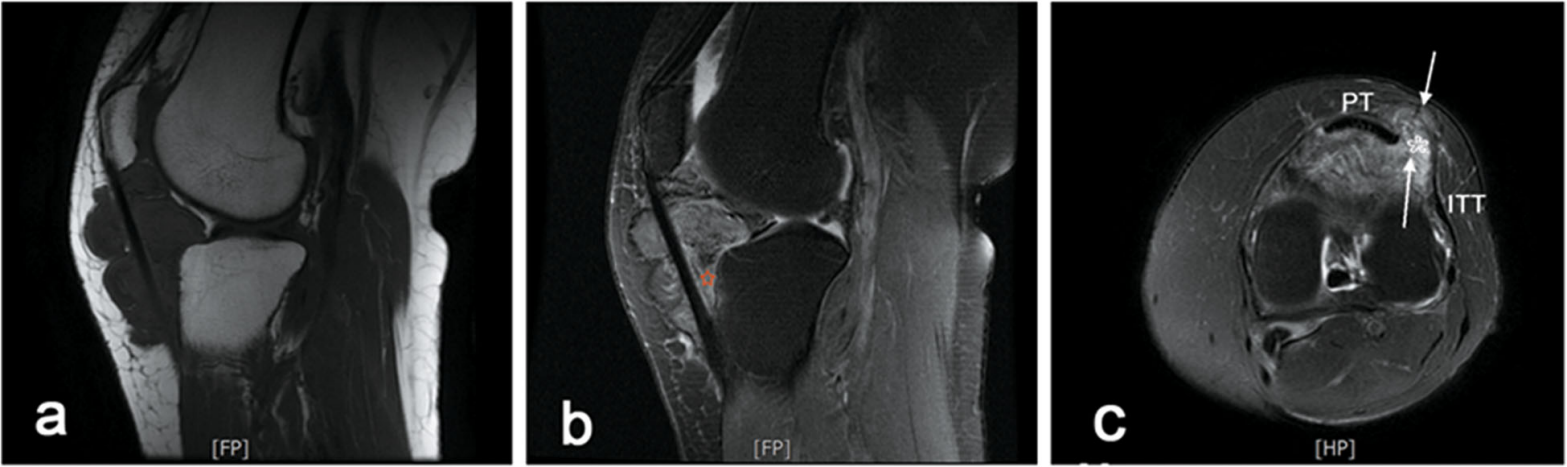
Preoperative MRI scans. a LPVNS manifested as a circumscribed and lobulated infrapatellar mass with an extra-articular extension through patellar tendon on sagittal T1-weighted images. b The pathognomonic appearance of PVNS, a “blooming artifact” caused by hemosiderin deposition (red pentastar), was observed on sagittal T2-weighted images. c Axial images revealed that extra-articular mass developed on both sides of the lateral patellar retinaculum, through a focal defect (asterisk), with a superficial component (arrowhead) and a deep component (arrowhead). ITT: Indicates iliotibial tract; PT: patellar tendon
